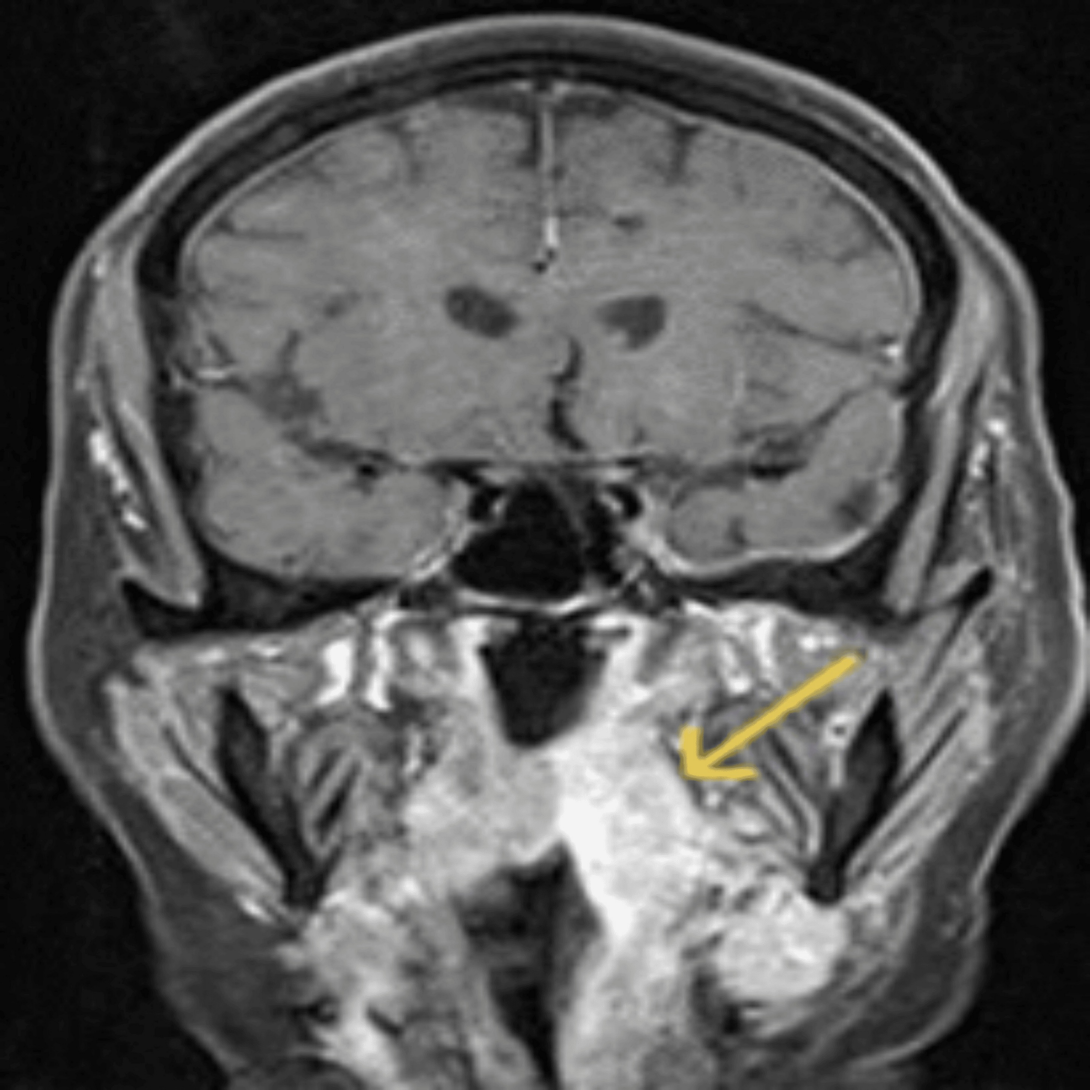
Coronal gadolinium-enhanced T1-weighted MRI The arrow indicates enhancement and thickening of the left-sided pharyngeal mucosal space.
